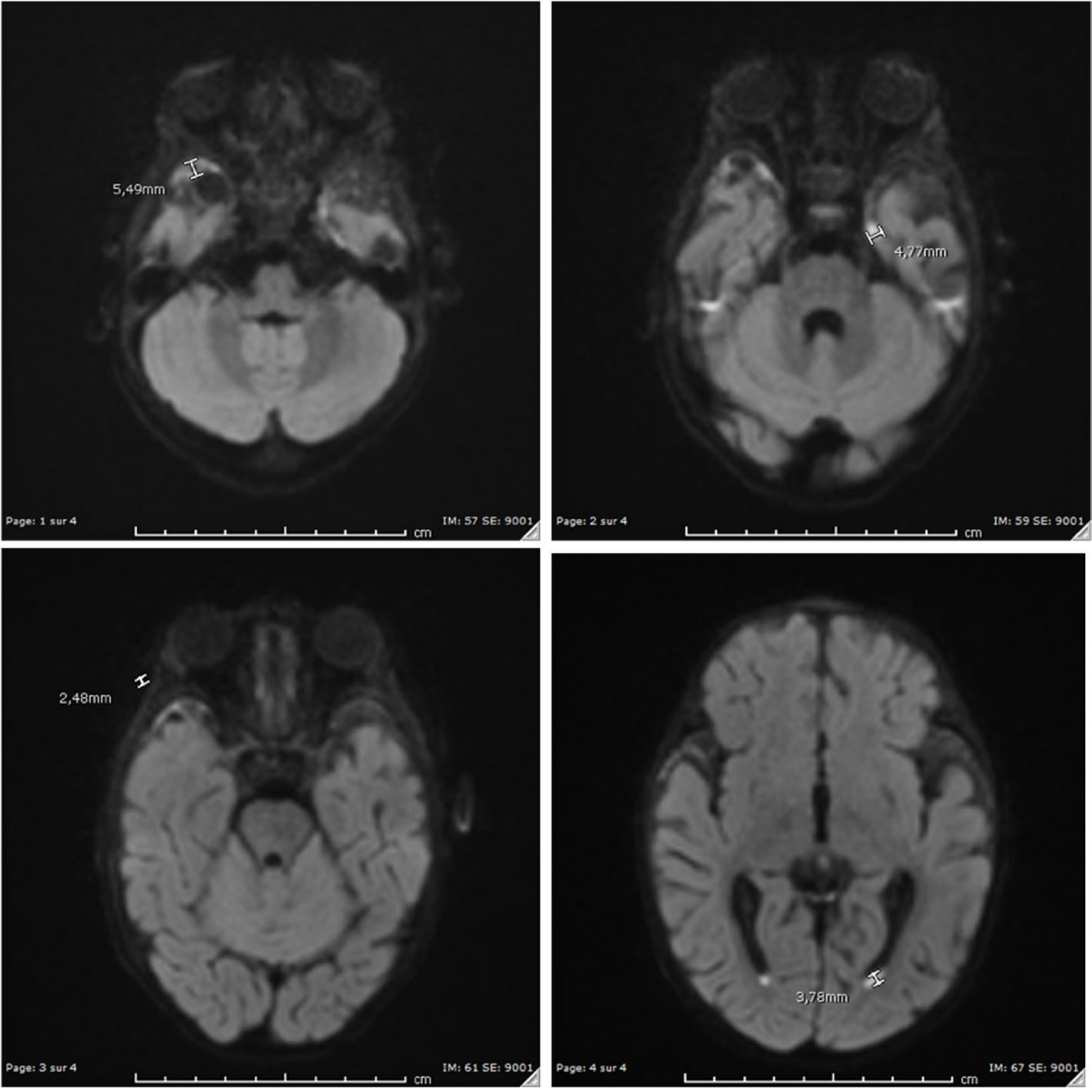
Brain magnetic resonance imaging showing purulent suffusion on day 2 during the first admission
